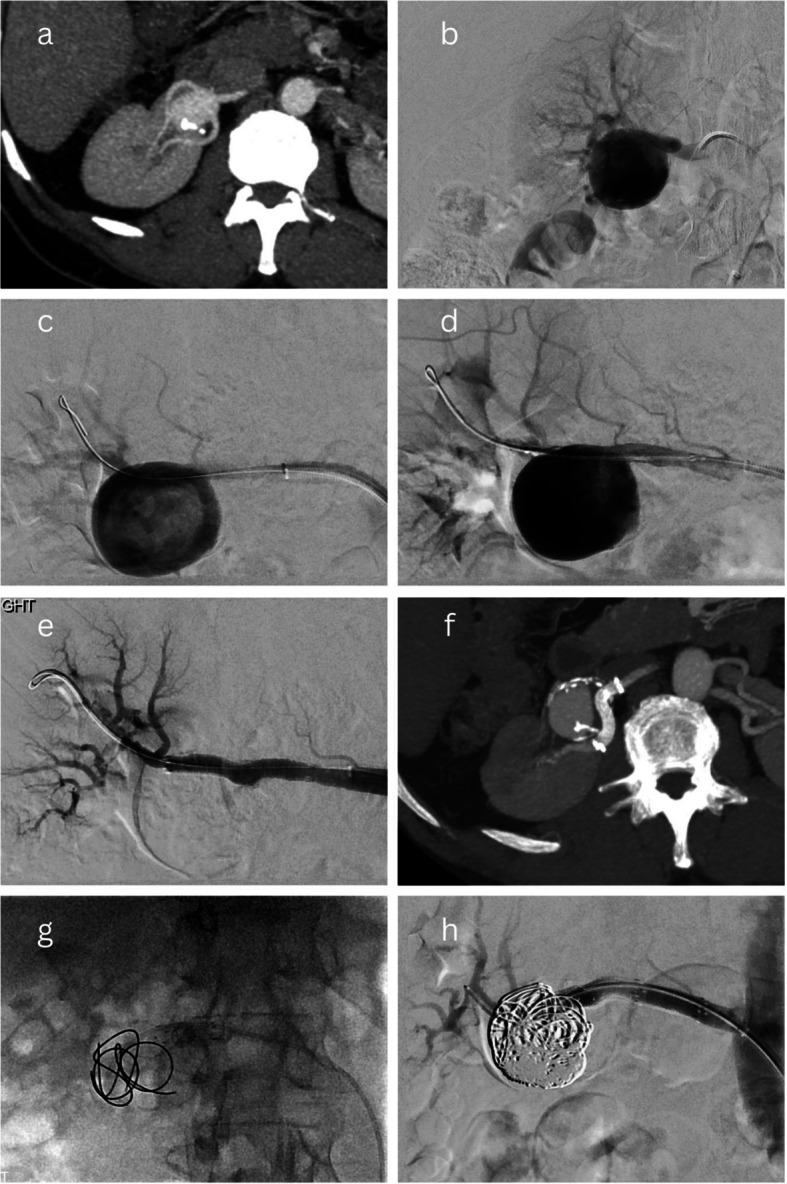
Stent-graft placement— Reintervention case 2. A 37-mm saccular Type 2 Rundback aneurysm with two efferent branches (a-b) was treated endovascularly by manipulating a wire across the aneurysm, into the posterior division efferent branch (c), and placing a covered stent across the aneurysm, successfully excluding it (d-e). Surveillance CT within the first year demonstrated partial reperfusion of the sac (f). At angiography, this was found to be due to an inadequate proximal seal and so a catheter was manipulated alongside the stent and into the aneurysm sac, which was subsequently embolised with coils (g), and the covered stent was extended proximally, successful excluding the aneurysm (h)
